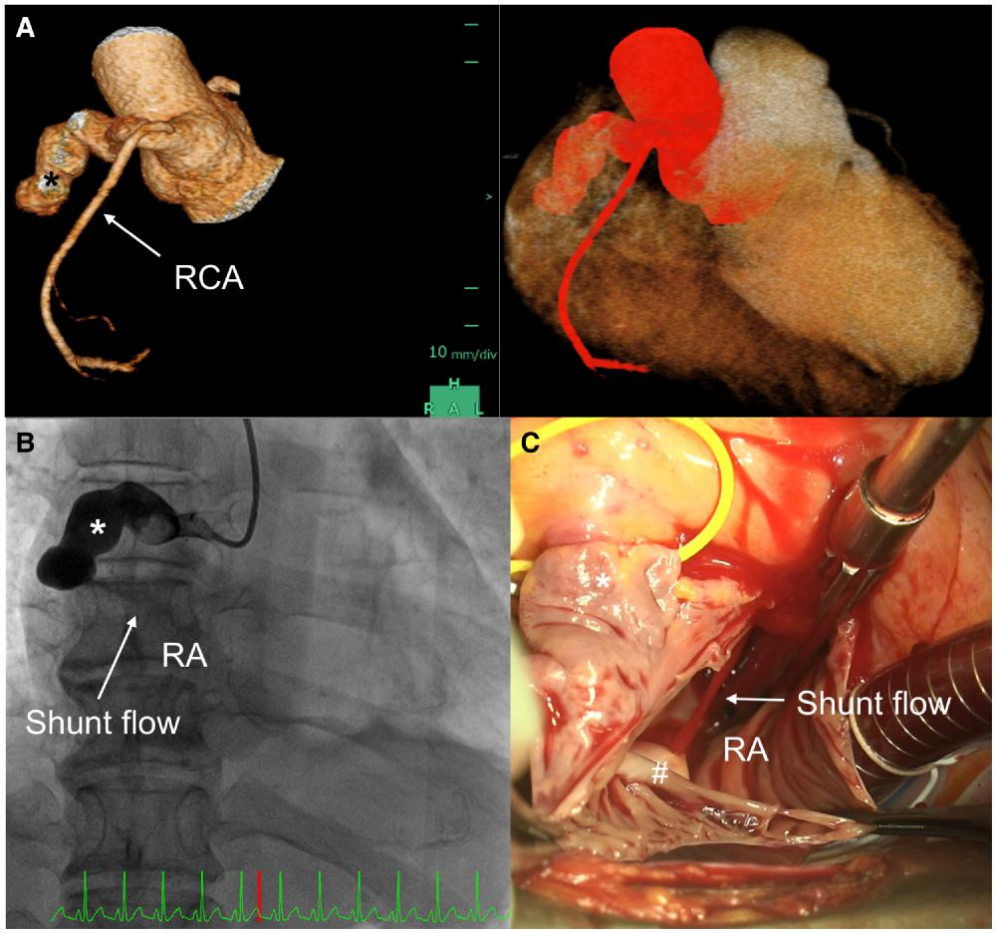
Morphology of the coronary artery fistula and turbulent shunt flow on computed tomography coronary angiography, coronary artery angiography, and a surgical image. (*A*) The morphology of the coronary artery fistula and right coronary artery on 3D computed tomography coronary angiography. (*B*) Coronary angiography showed the coronary artery fistula (asterisk), shunt flow from the coronary artery fistula, and contrast-enhanced right atrium. (*C*) Surgical findings revealed the enlarged coronary artery fistula (asterisk) and shunt flow from the outlet of the fistula (number sign). RA, right atrium; RCA, right coronary artery.
